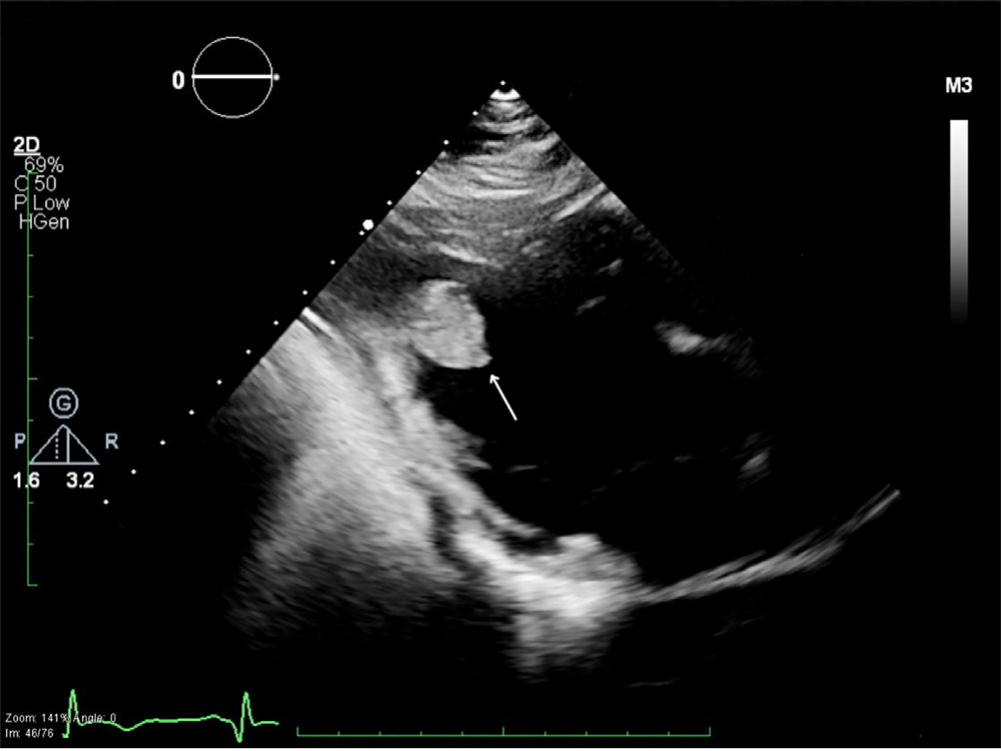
Echocardiographic report demonstrating LVEF 46%, regional wall motion abnormalities, and LV thrombus (arrow).
